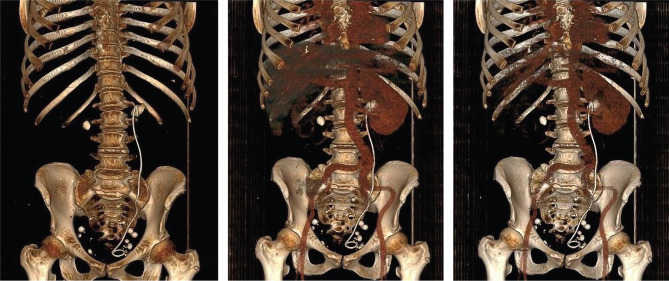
CT 3D reconstruction of bilateral stone disease located in the renal pelvis and left double J stent placement.
